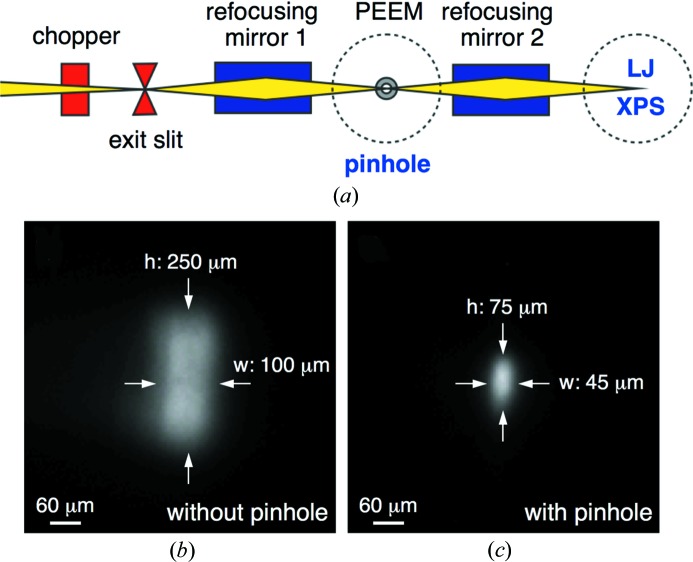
SIM beamline layout (*a*) and X-ray spot image after the second toroidal refocusing mirror: (*b*) without a pinhole and (*c*) with a 30 µm pinhole in the intermediate focus of the beamline. In both images the exit slit of the beamline is 100 µm and the photon energy is 800 eV.
